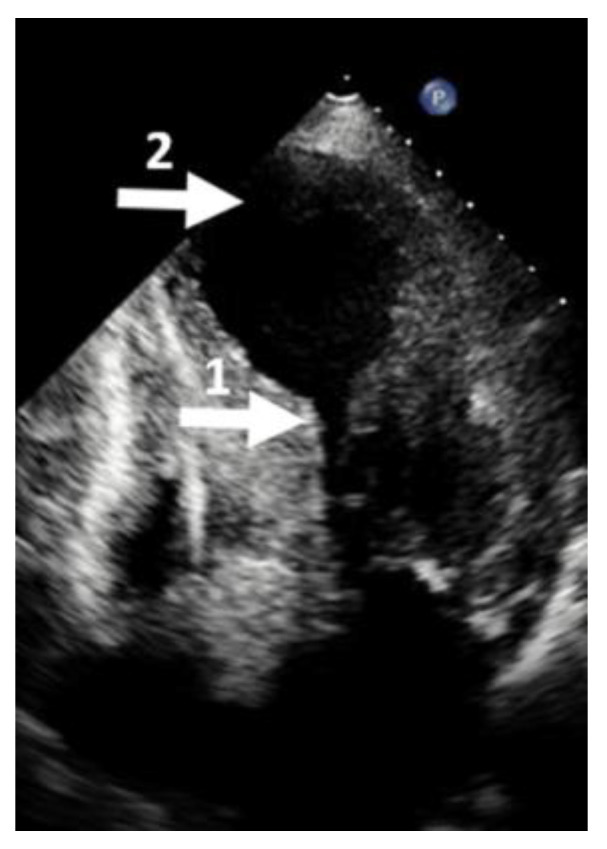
Echocardiographic four-chamber view. (1) Mid cavity obliteration; (2) left ventricular aneurysm. This picture is called the hour-glass appearance.
